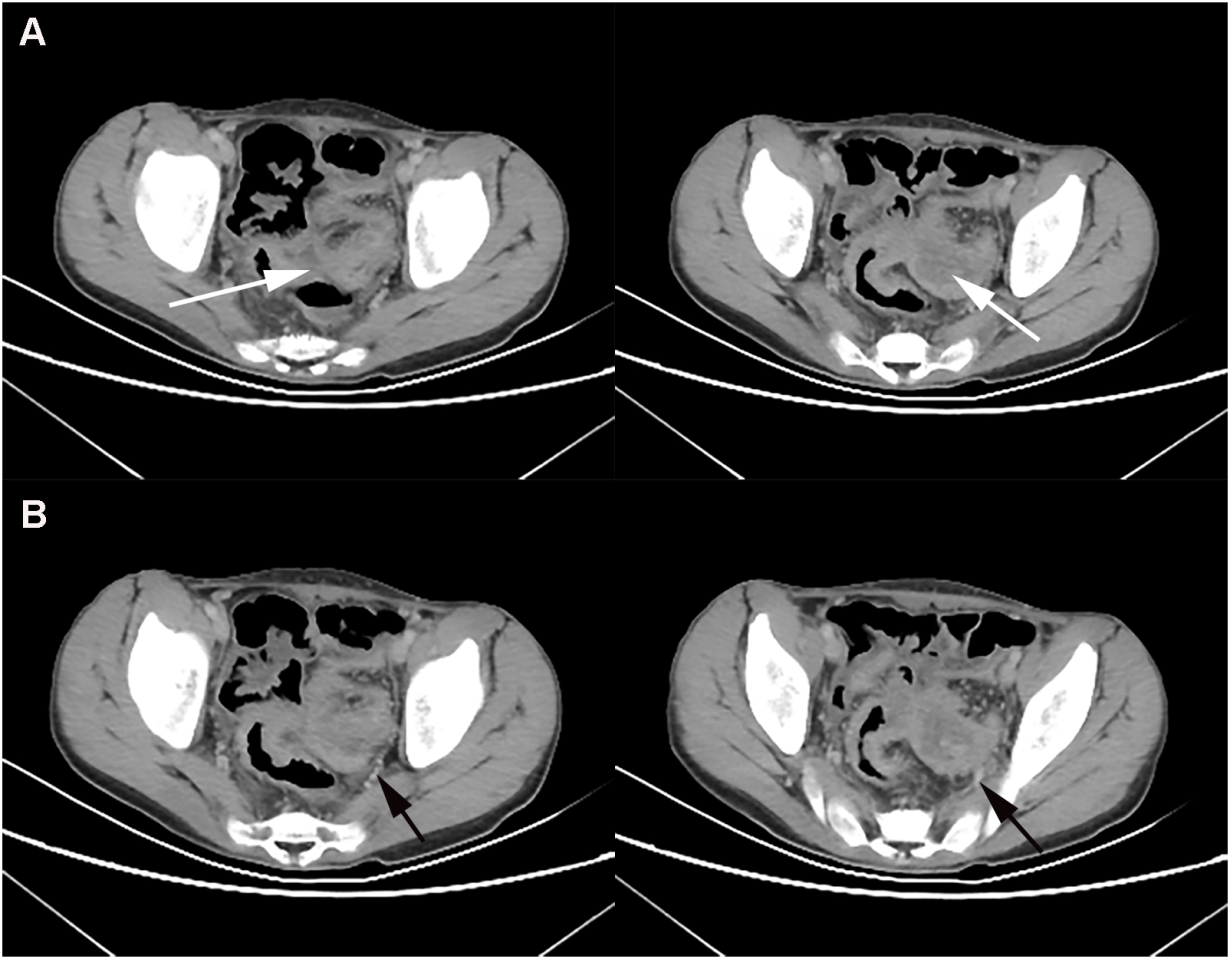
At baseline (November 2021), contrast-enhanced CT showed thickening of the wall of the upper rectum and part of the sigmoid colon with significant narrowing of the intestinal lumen (A), and the lesion was closely associated with the left iliac vessels (B).
